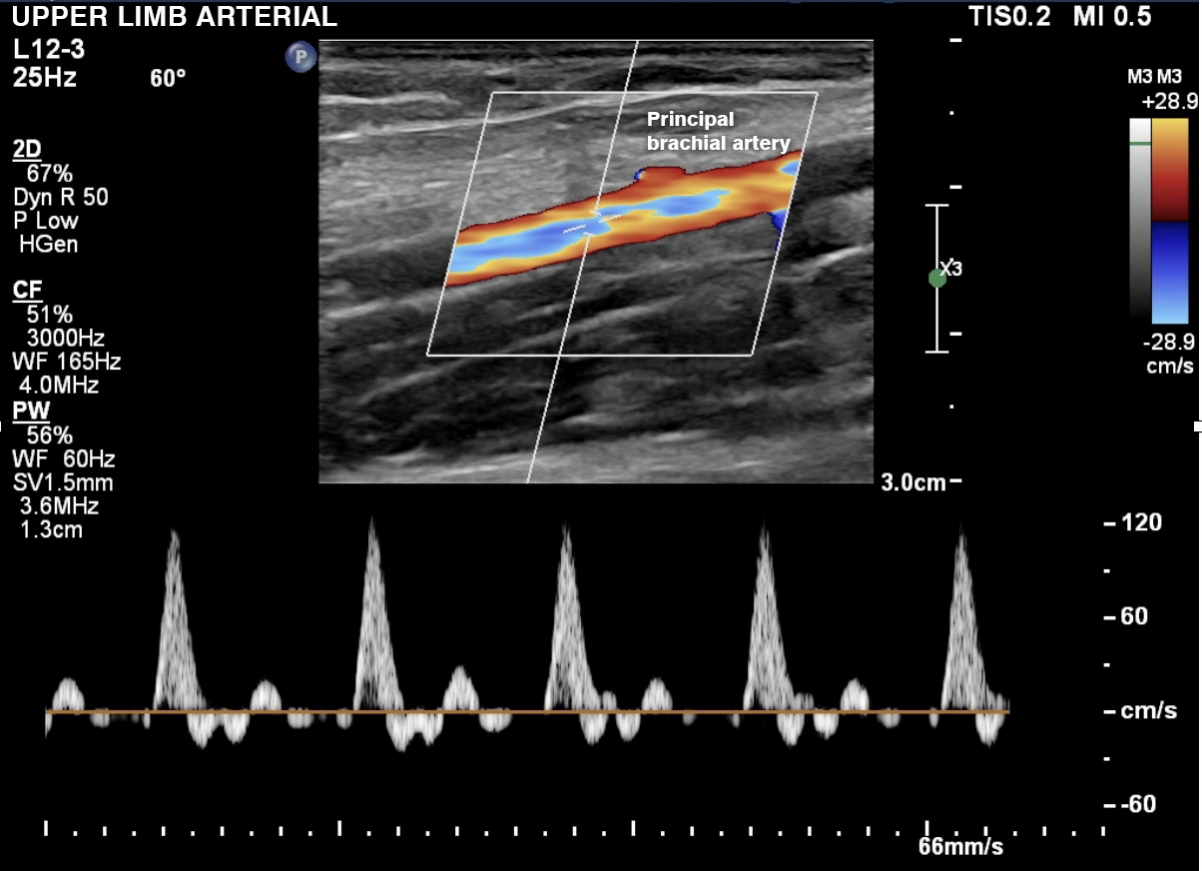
Doppler ultrasound in spectral mode, longitudinal view, showing normal flow in the brachial artery.
